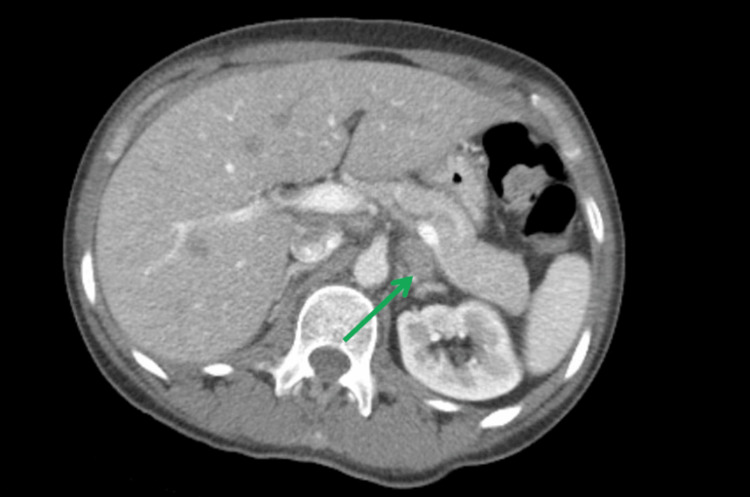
CT abdomen/pelvis with contrast demonstrating enlarged lymph nodes (green arrow) in the upper abdomen.
